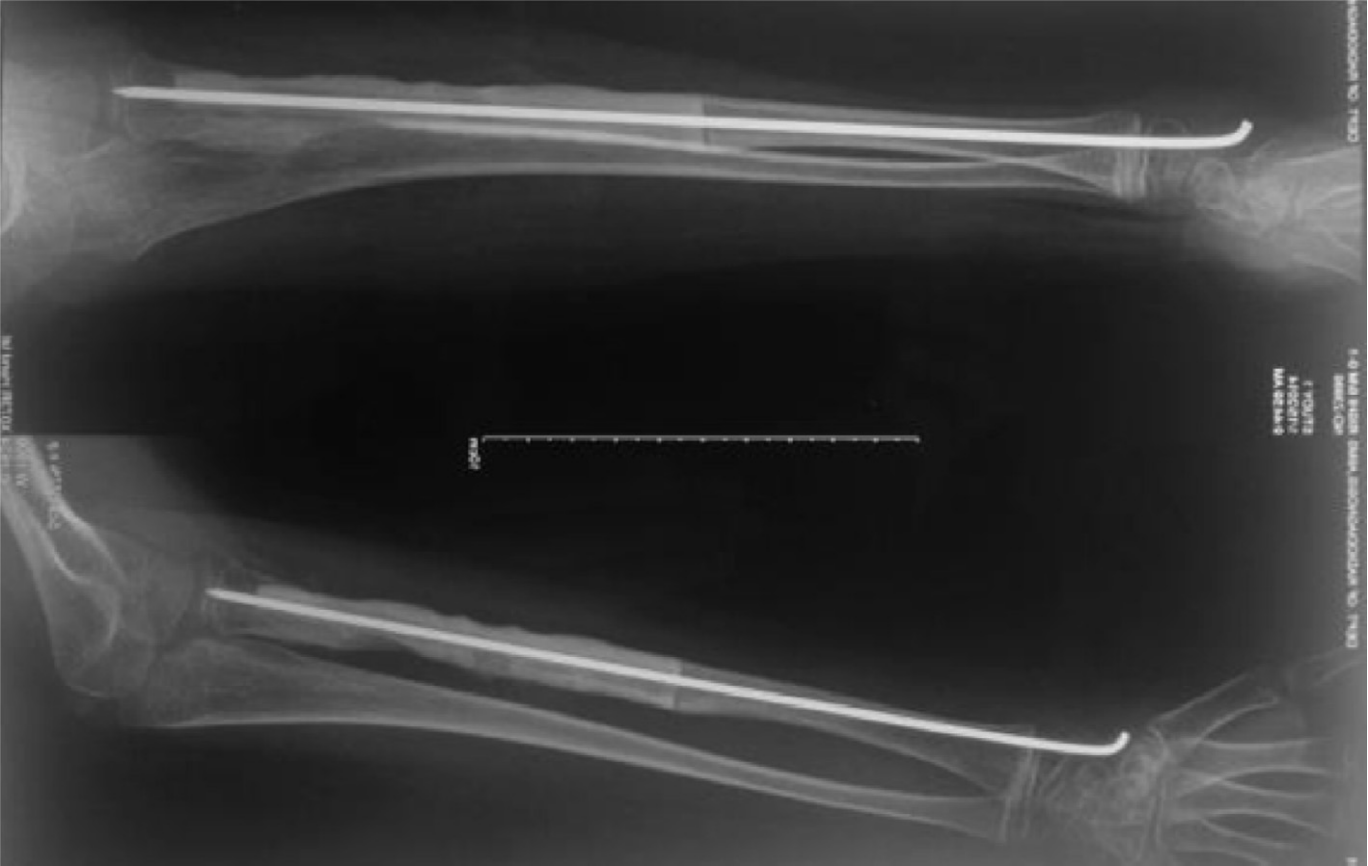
Twelve weeks follow-up X-ray showing incorporation of the graft at the parent-allograft bone interface; resorption of cortical margins is visible. 35 mg of alendronate was started at this time.
